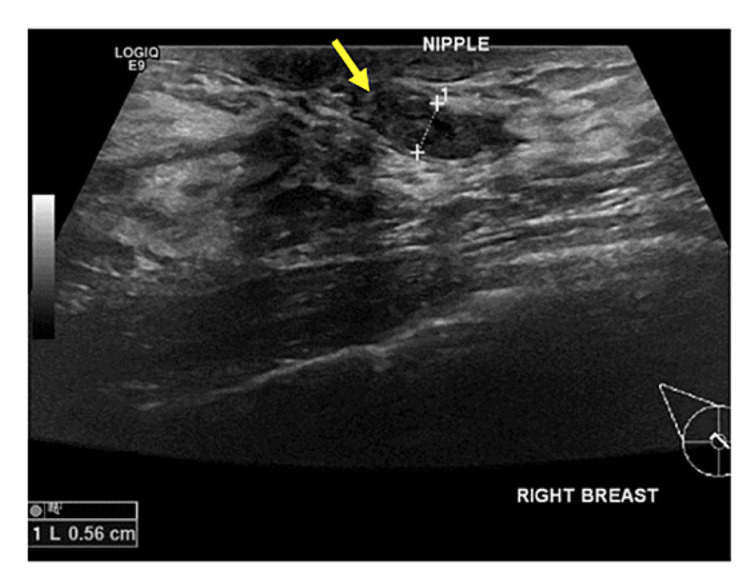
Pathology-proven papilloma with ultrasound findings of dilated retroareolar duct with an echogenic mass within (yellow arrow).
